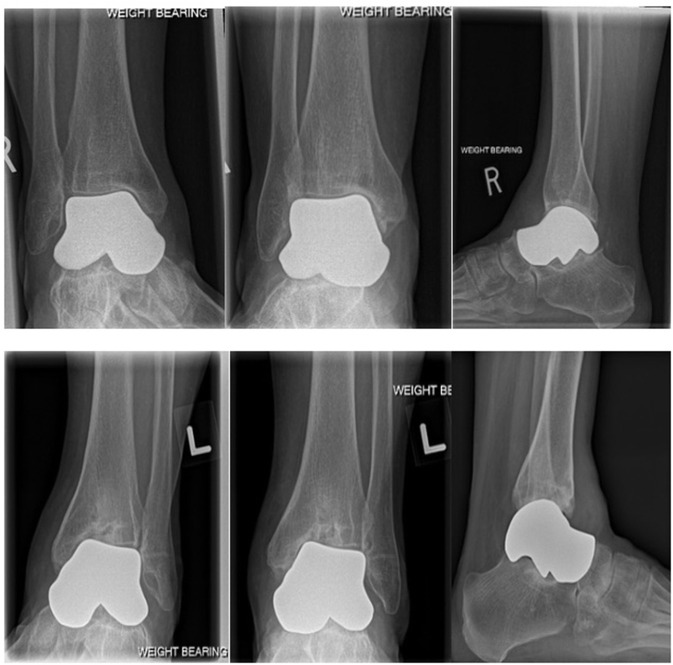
Top row: Weightbearing radiographs (mortise, AP and lateral) 34 months post-operative right total talus replacement. Bottom row: Weightbearing radiographs (mortise, AP and lateral) 1 year post-operative left total talus replacement. As noted in the preoperative radiograph, the medial corner of the tibial plafond was compromised resulting in tilting of the prosthesis in the ankle mortise.
